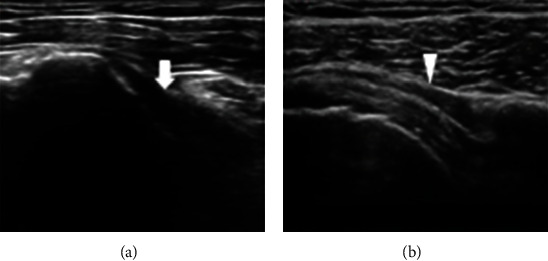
Ultrasound images of SC bursa in healthy (a) and bursitis in painful (b) shoulders. Subcoracoid bursa in healthy shoulder (arrow). In subcoracoid bursitis, thickened bursa with effusion can be observed (arrowhead).
